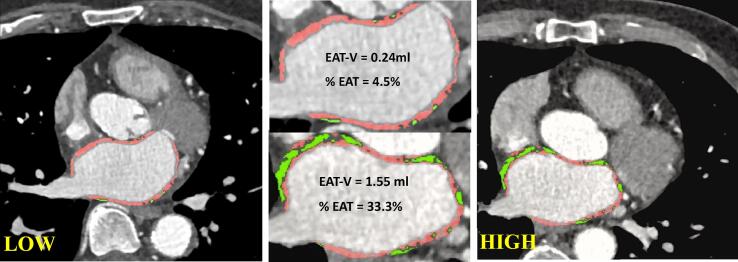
Left and upper middle: low EAT% in the LA wall (EAT-V = 0.22 ml, MYO-V = 4.63 ml, LA volume measured by TTE = 103 ml, EAT%=4.5 %). Right and upper middle: high EAT% in the LA wall (EAT-V = 1.55 ml, MYO-V = 3.11 ml, LA volume measured by TTE = 43 ml, EAT%=33.3 %). EAT-V = epicardial adipose tissue volume; MYO-V = myocardial volume; LA = left atrial; TTE = transthoracic echocardiogram.
